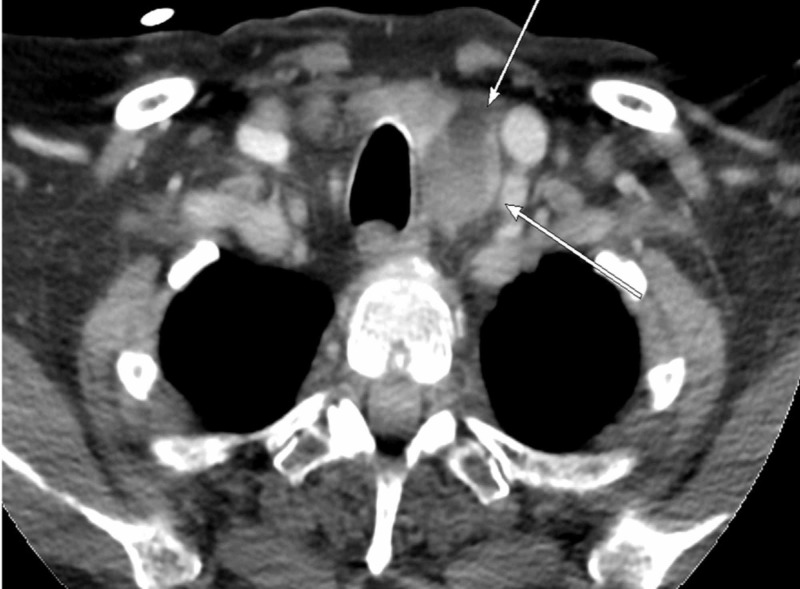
Axial section of parathyroid 4 dimension (4D) computed tomography (CT) demonstrating abnormal mass abutting the posterior left aspect of the thyroid gland representing a parathyroid adenoma
